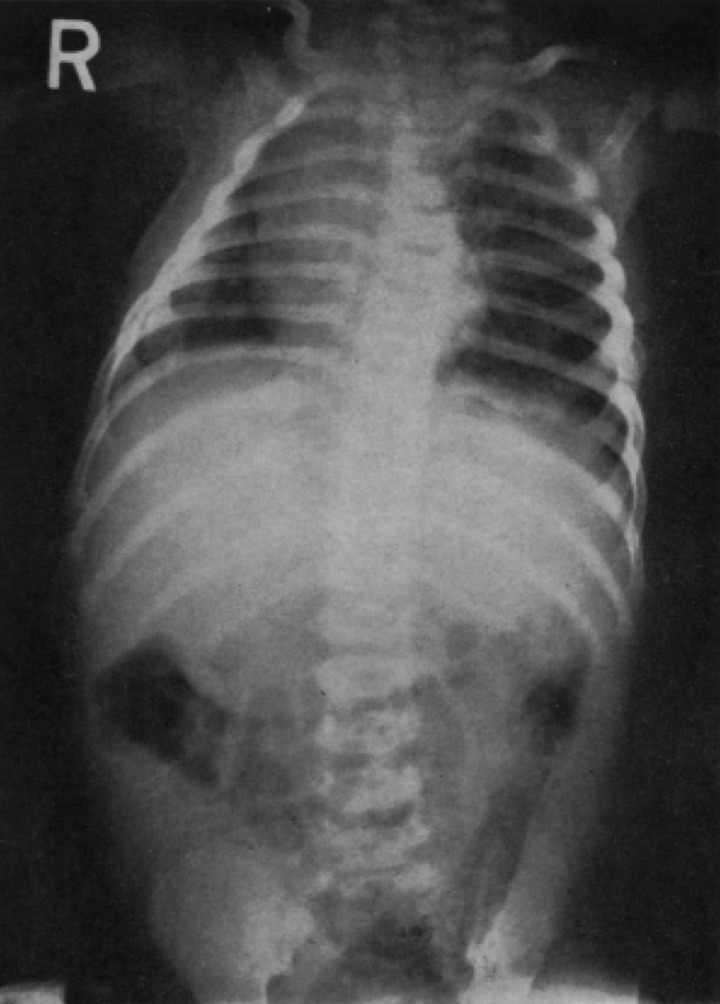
X-ray showing healing fractures in seven ribs of a two-month-old baby.
